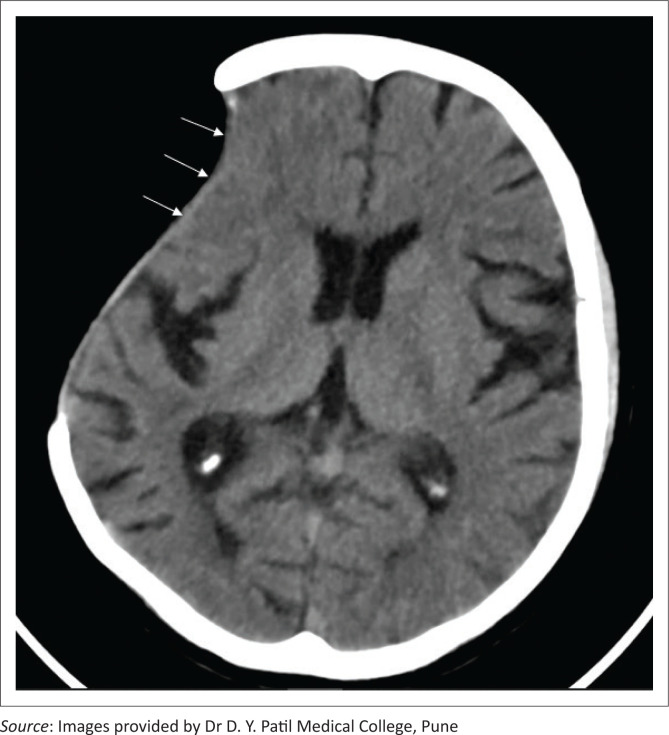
Trephine syndrome. Axial soft tissue window CT brain image reveals a right fronto-parieto-temporal craniectomy with a sunken skin flap (white arrows) and mild deformation of the underlying brain parenchyma without midline shift.
